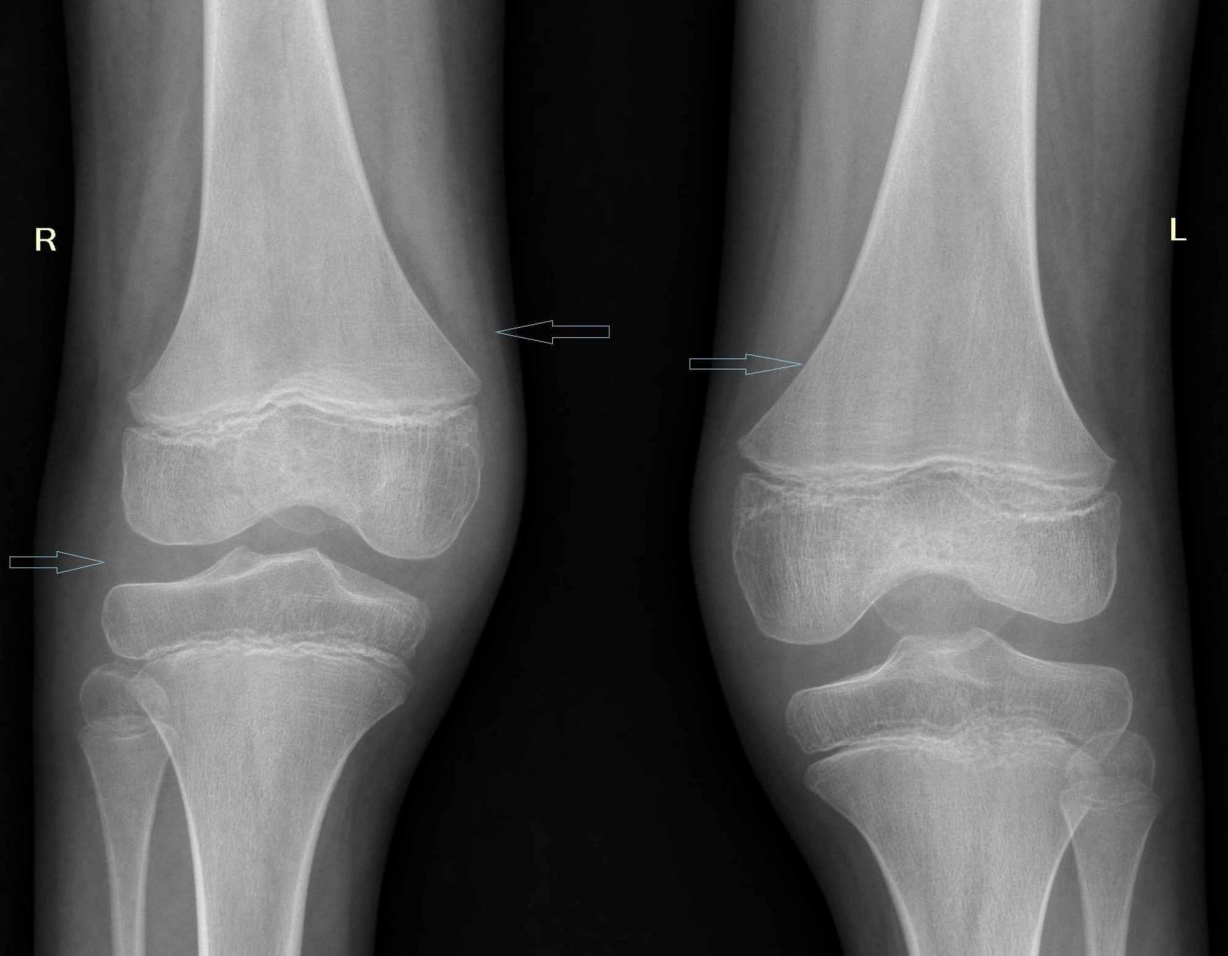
Bilateral knee joint X-ray showing soft tissue swelling with mild osteopenia
